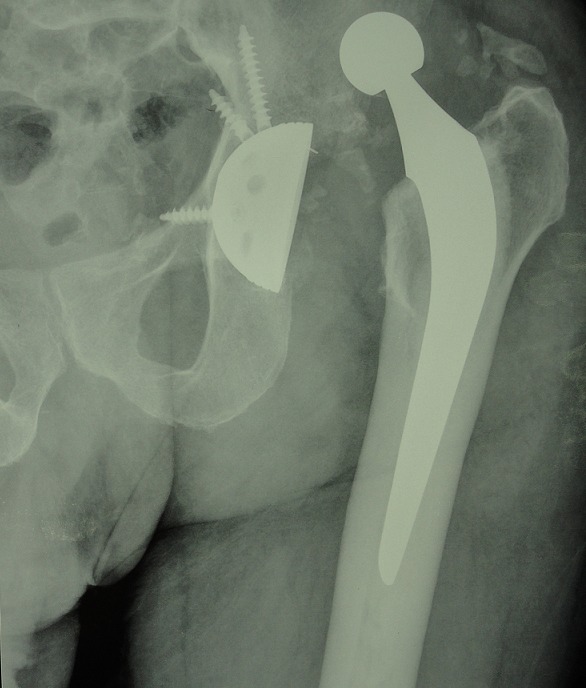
Radiographie standard de la hanche montre une luxation de prothèse totale de la hanche, la malposition de cotyle constitue la cause principale
